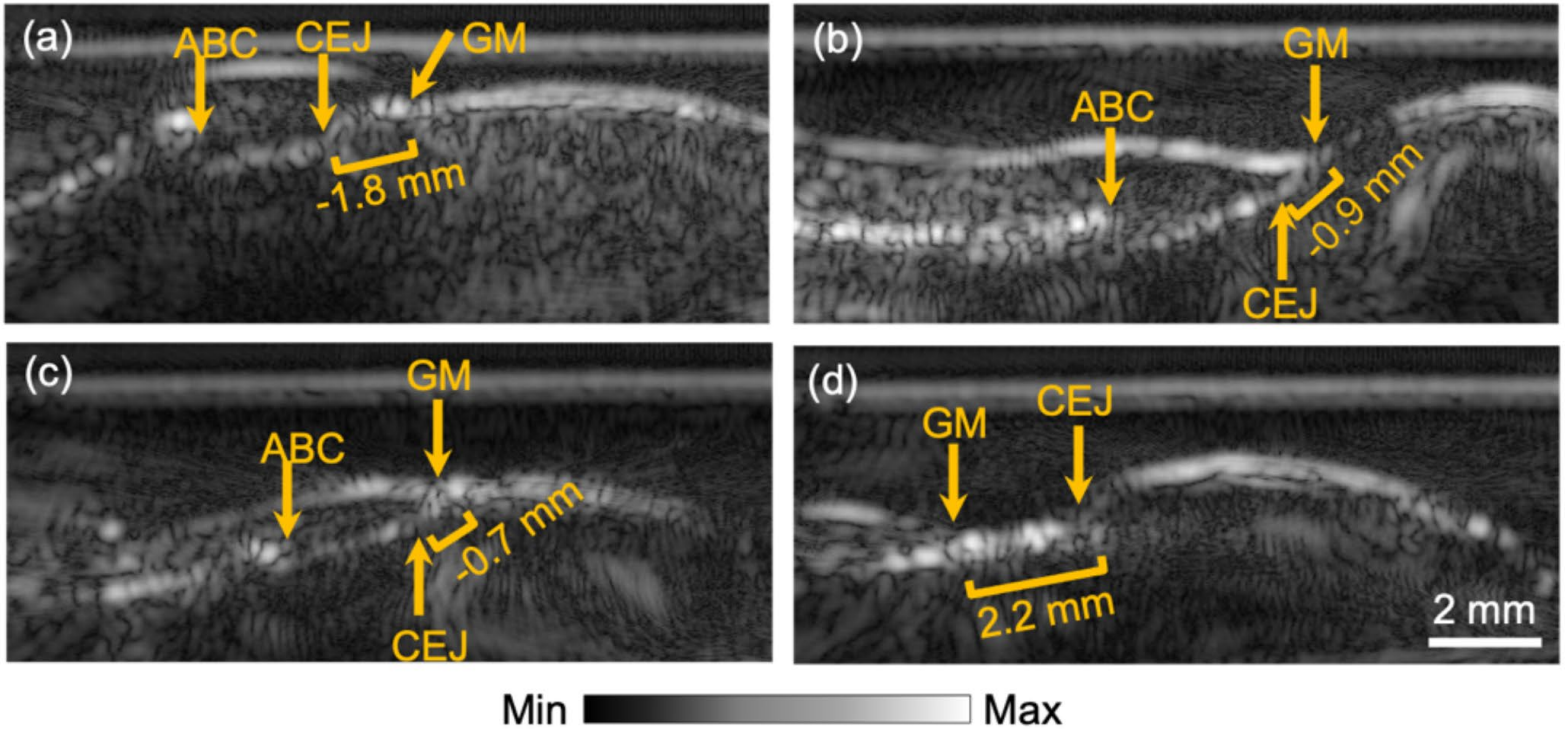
Four typical tooth images showing different levels of gingival recession. (a) An ultrasound image of incisor #8. (b) 1st molar #30. (c) 1st premolar #12. (d) Canine #6. The imaging-based gingival recession (iGR) were − 1.8 mm, -0.9 mm, -0.7 mm, and 2.2 mm for (a), (b), (c), and (d), respectively. Note that the value of iGR is positive in clinical recession, as shown in (d)
